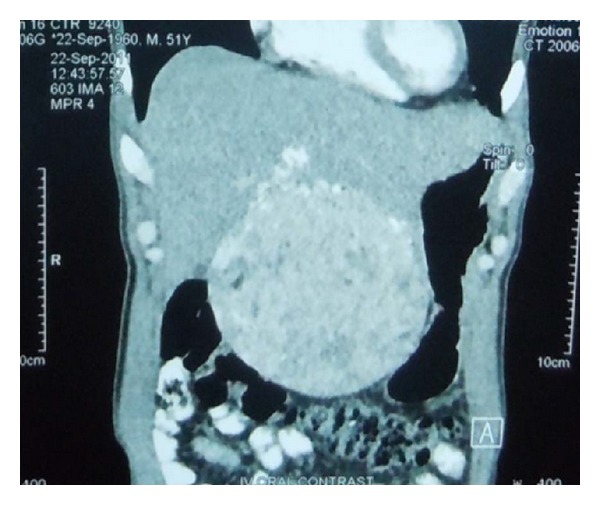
CT liver showing a mass lesion.
